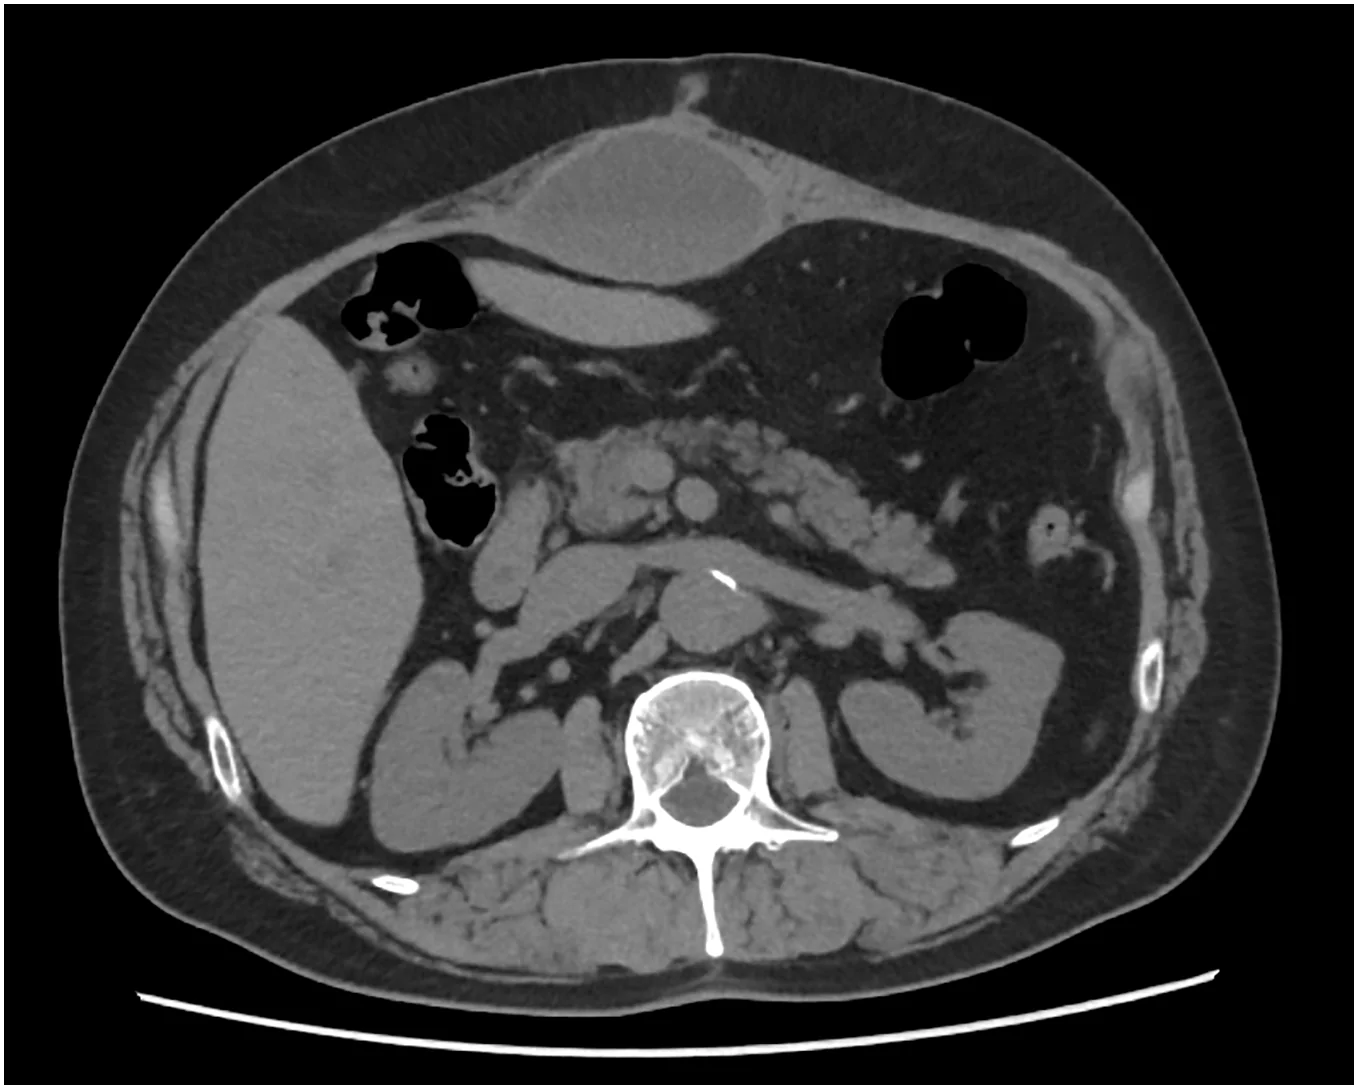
Preoperative CT findings of a chronic encapsulated seroma after Rives–Stoppa repair. Axial CT image obtained 6 months after retromuscular incisional hernia repair demonstrating a large encapsulated fluid collection interposed between the rectus muscle and the retromuscular mesh, without surrounding inflammatory enhancement or evidence of mesh infection.
